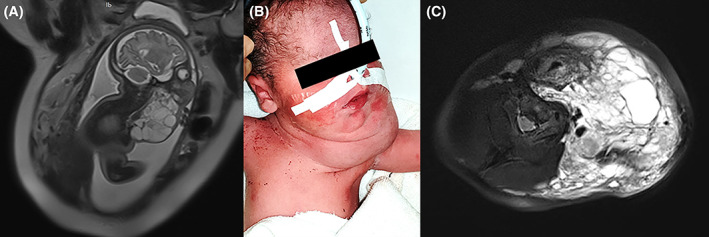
(A) Fetal MRI showing a large lymphatic malformation with high suspicion of airway compromise (deviation of the airway, floor of mouth and base of tongue involvement). (B) newborn intubated after the EXIT procedure. (C) axial MRI view of the lymphatic malformation at 3 months of age with deviation of the airway to the right. Despite massive cervical involvement, the case was managed without the need for a tracheotomy
